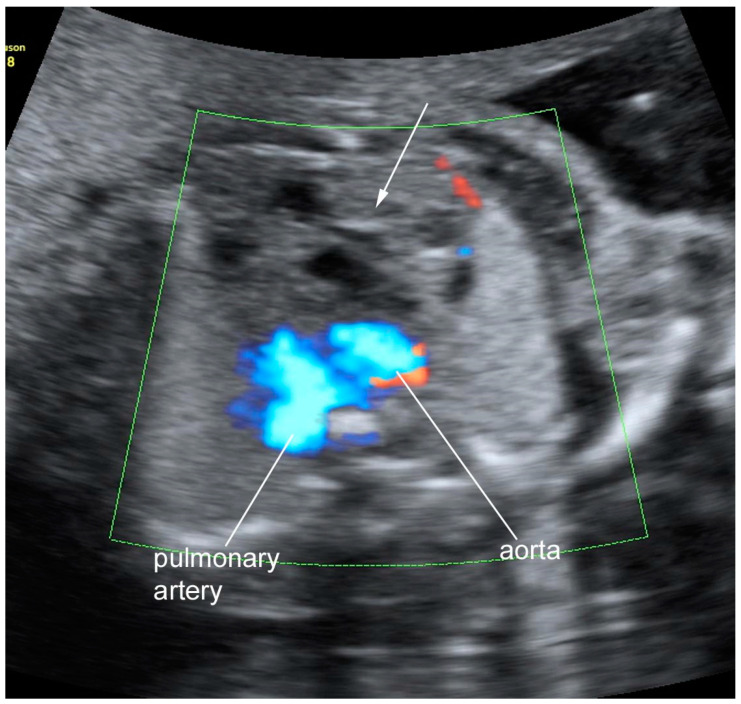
Ultrasound image of the teratoma: transverse view of the fetal chest at 22 weeks; a complex mass with solid and liquid components was seen in the upper and anterior part of the thorax (arrow).
